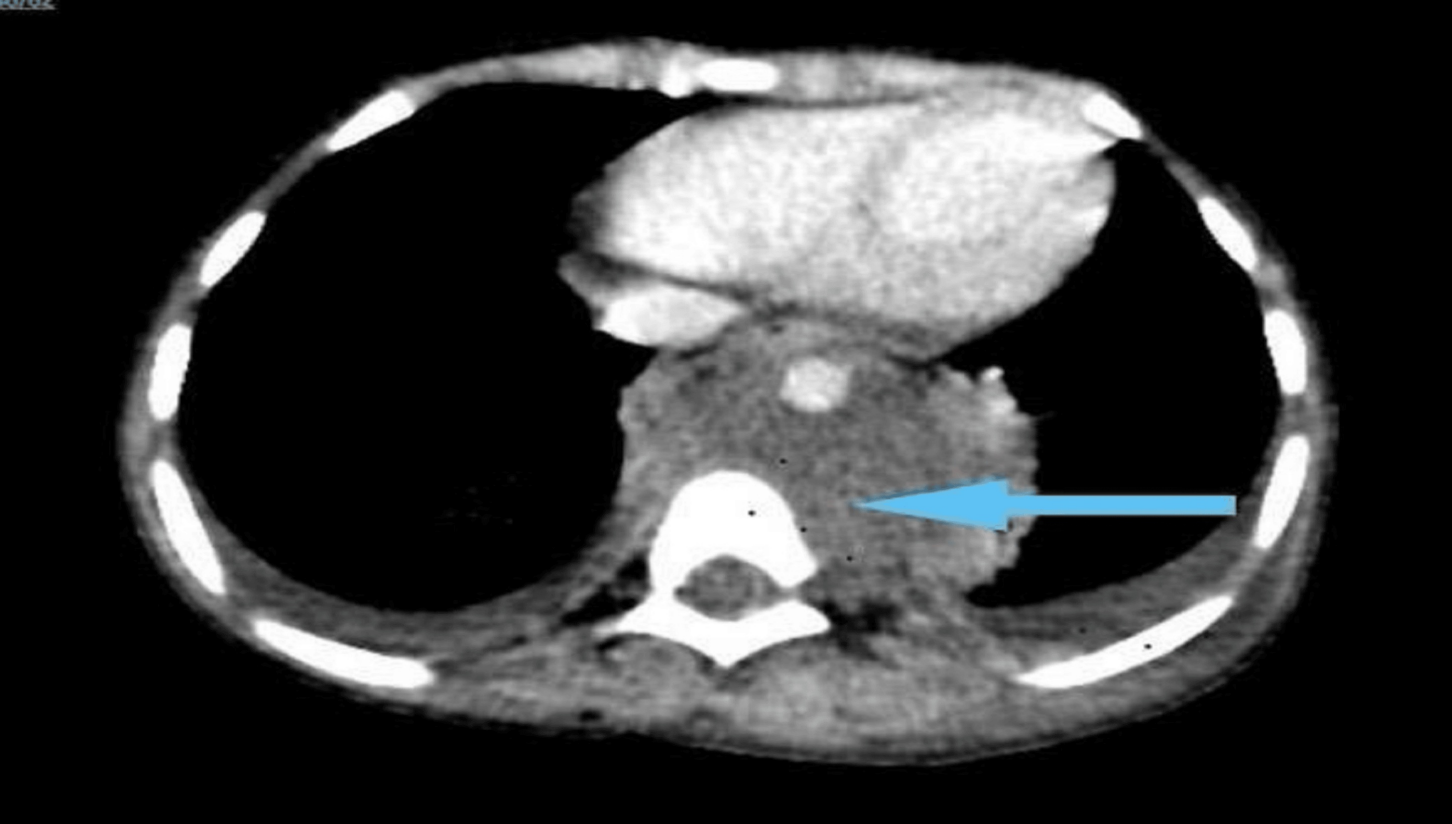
CT scan showing retroperitoneal and retroaortic soft tissue mass.
